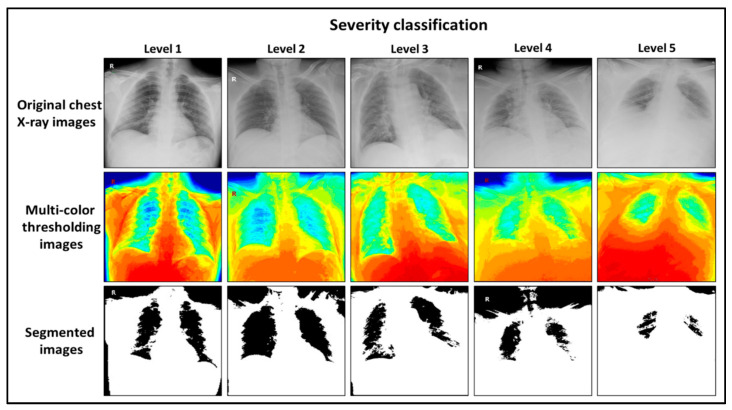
Chest X-ray images for five confirmed SARS-COV2 cases. These images show the levels of severity of inflammation in three different modes: original image mode (first row), multi-color thresholding mode (second row), and segmentation mode (third row).
